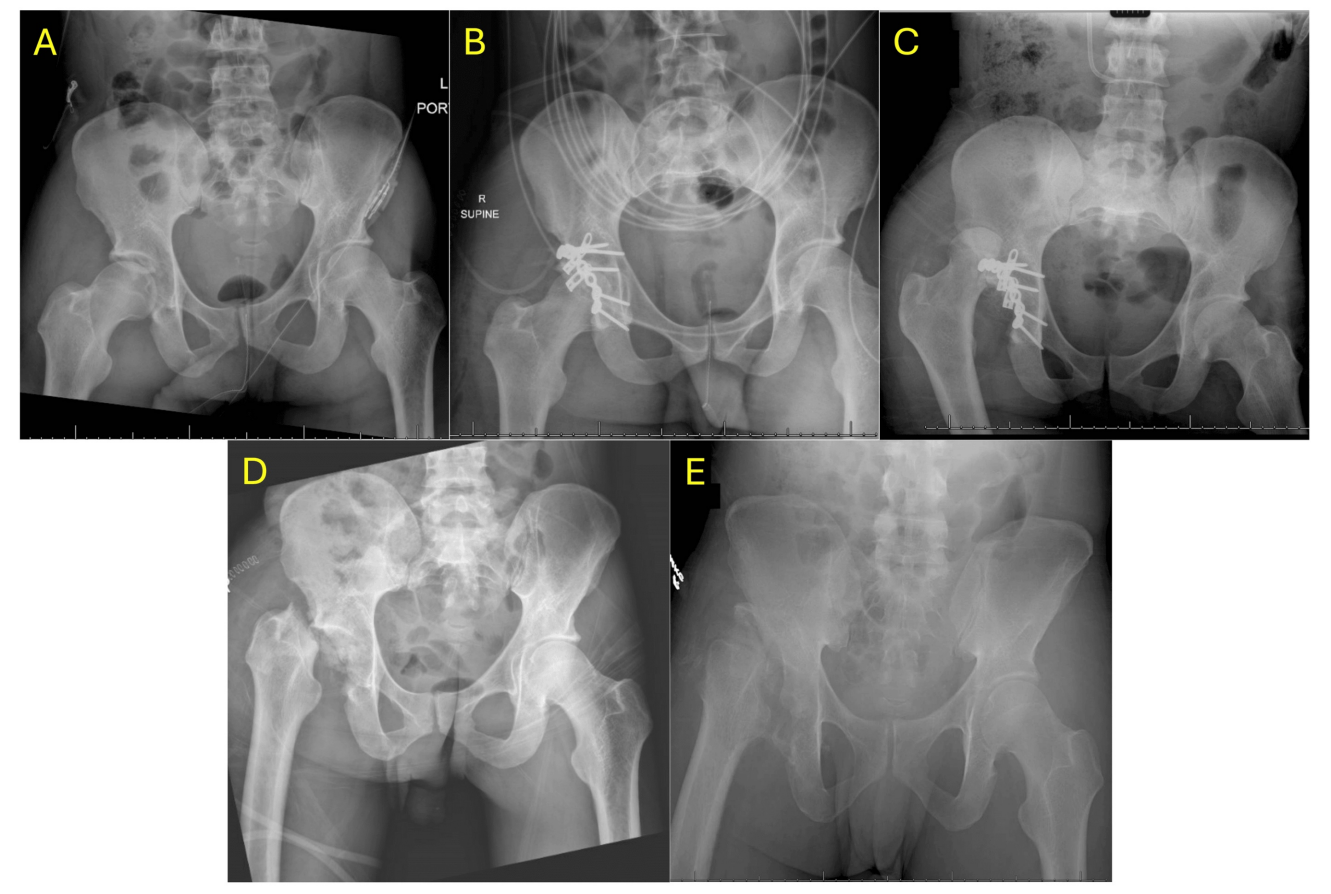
Serial radiographs of subject 1. (A) Injury film demonstrating initial fracture pattern. (B) Immediate post-operative radiograph following index fixation. (C) Radiograph obtained 26 days post-index surgery, prior to Girdlestone resection. (D) Immediate post-operative radiograph following the Girdlestone procedure. (E) Radiograph obtained 180 days post-index surgery demonstrating the post-operative course.
